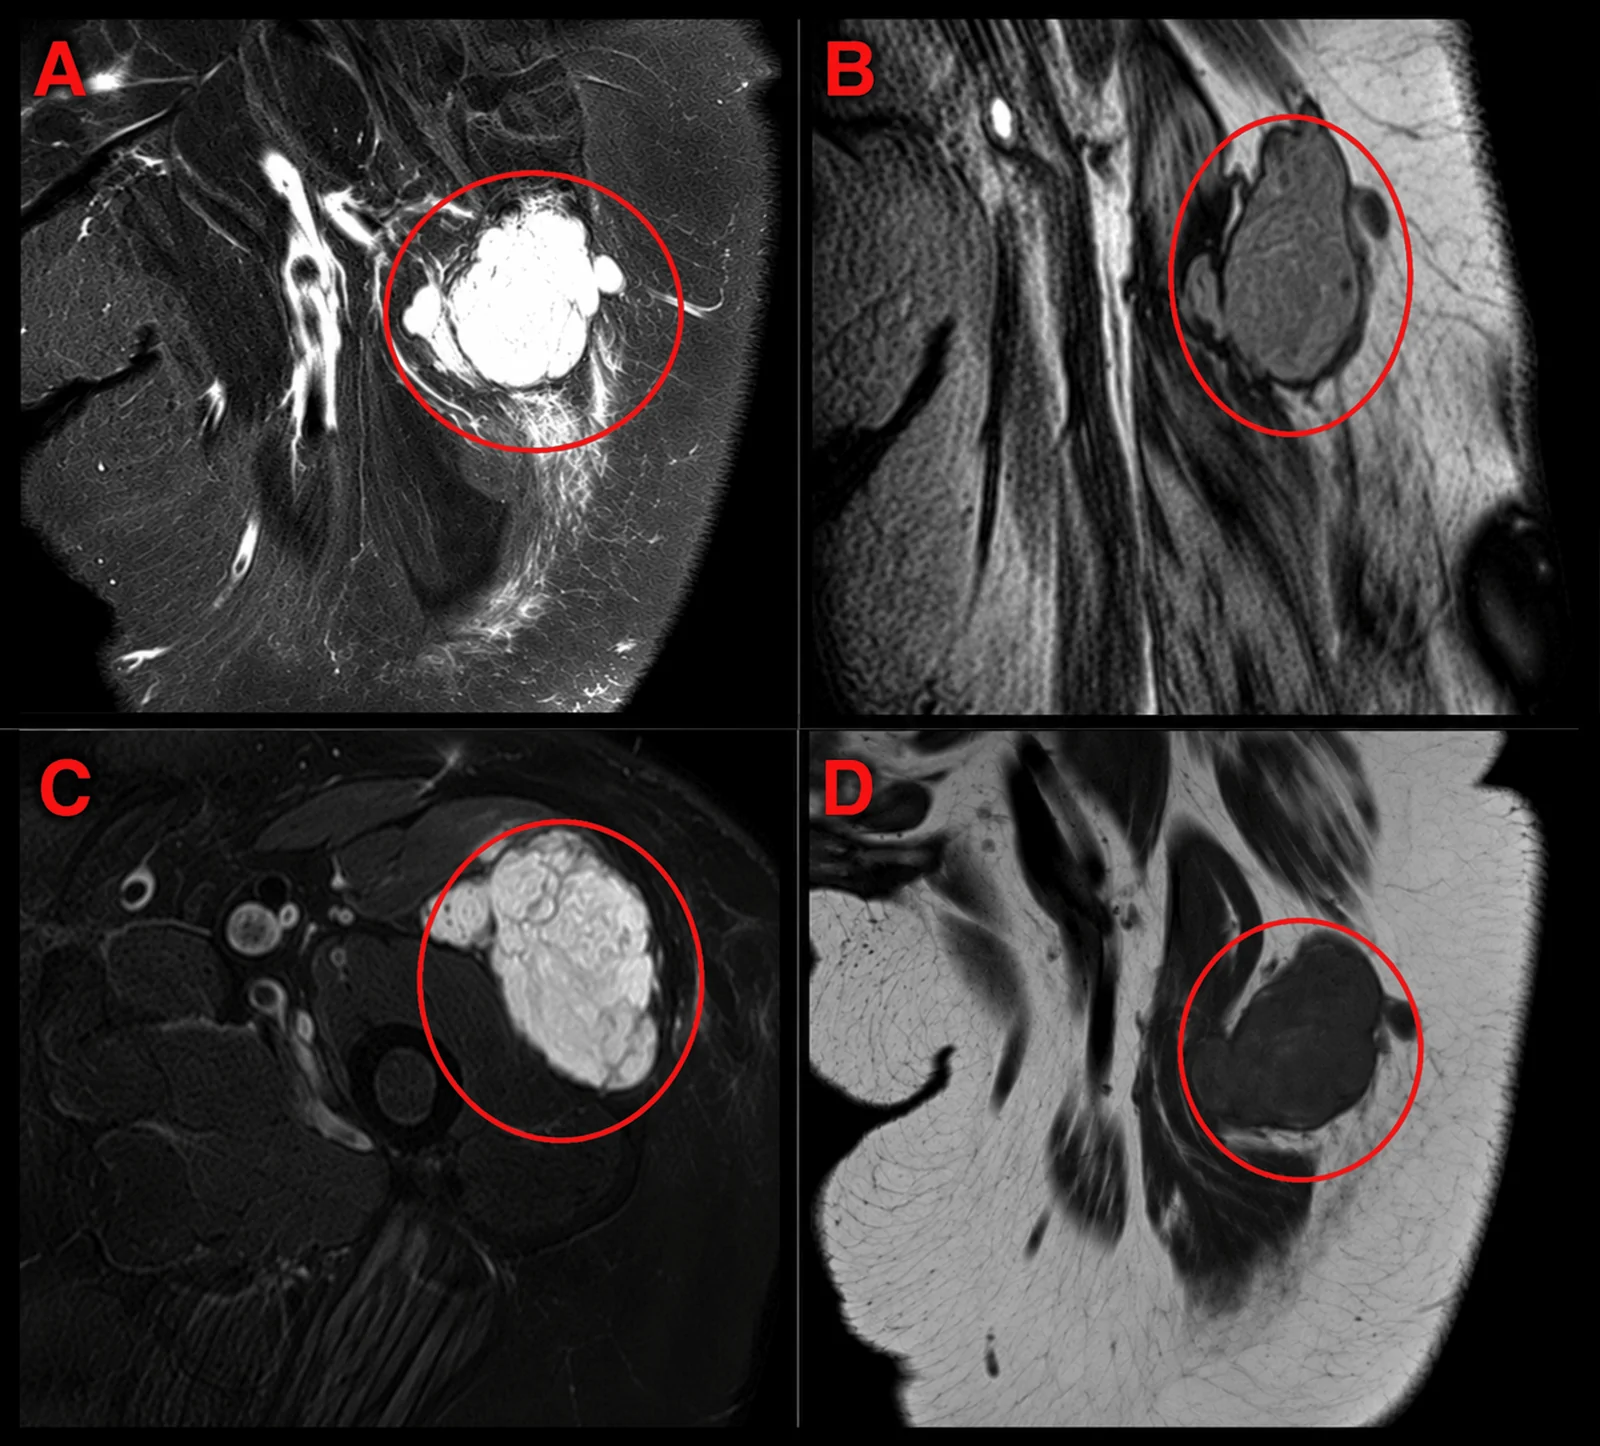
(A) Coronal T1-weighted post-contrast showing a well-circumscribed lesion (red circle) with heterogeneous enhancement. (B) Sagittal view demonstrating an ovoid, relatively homogeneous mass (red circle). (C) Axial fluid-sensitive sequence revealing a heterogeneous, hyperintense lesion with internal complexity and surrounding soft tissue edema (red circle). (D) Coronal T1-weighted pre-contrast image confirming the intramuscular location and well-defined margins of the lesion (red circle).
